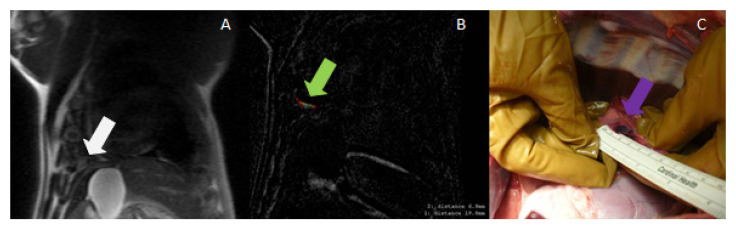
(A) MRI (localizer) used for treatment planning in the pleura (white arrow); (B) Post-ablation MRI (subtraction of LAVA pulse sequence images before and after ablation), showing a very small ablated area near the diaphragm (green arrow); (C) necropsy image showing the ablated area in the pleura (purple arrow).
